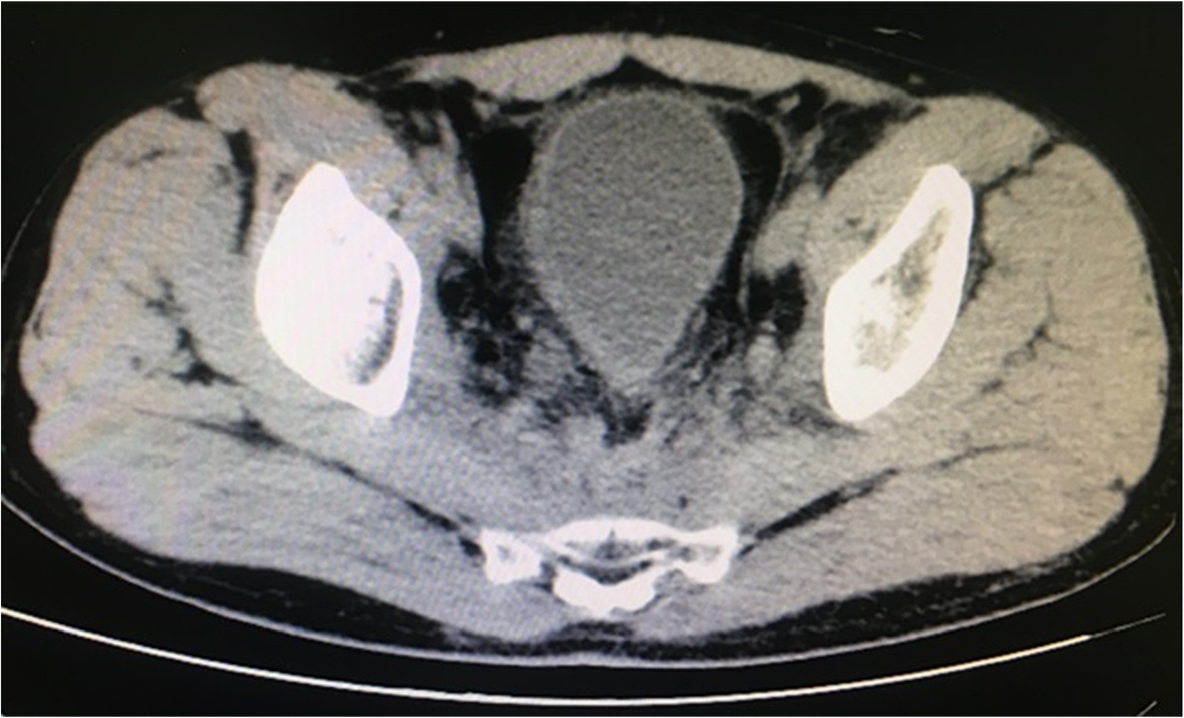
Altered bladder shape after radical resection of rectal carcinoma, shown by computed tomography
